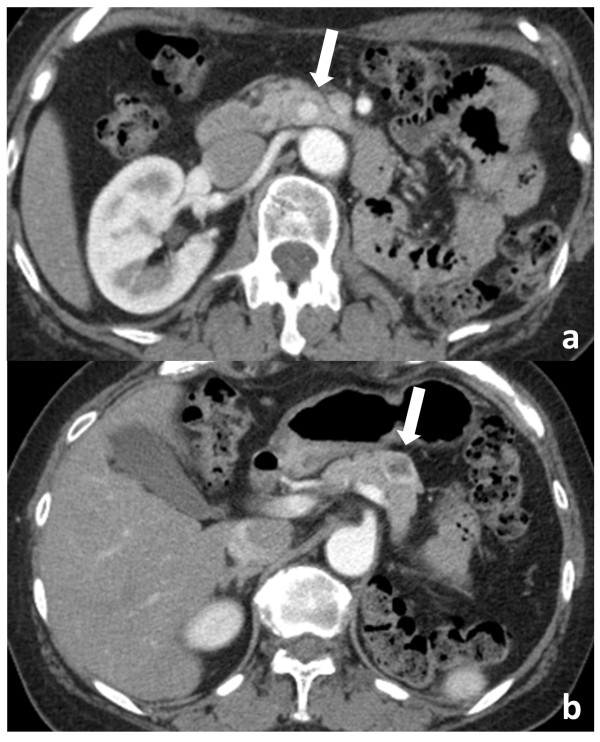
CT findings of the pancreatic metastases (arrows). a. Homogenous hypervascular head lesion. b. body lesion with central necrosis and enhanced rim.
